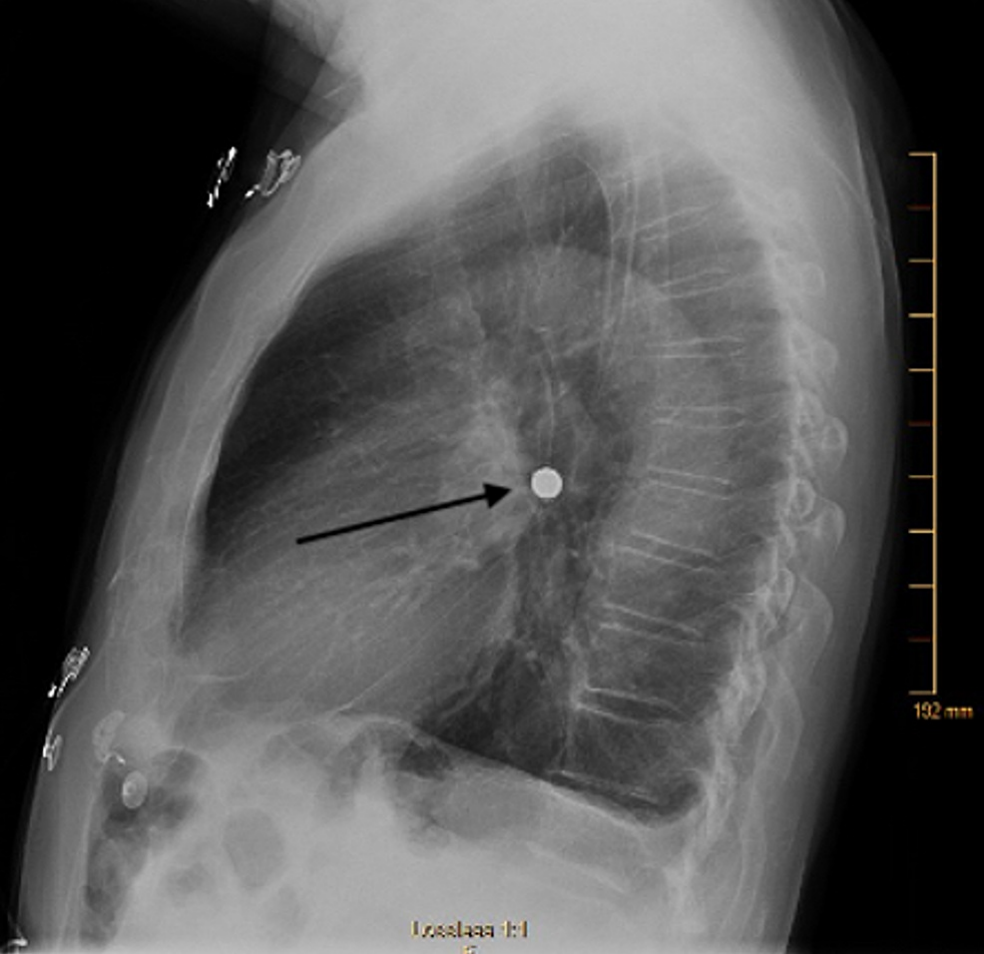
Lateral view of chest x-ray, which was obtained after live viewer demonstrated bronchus
